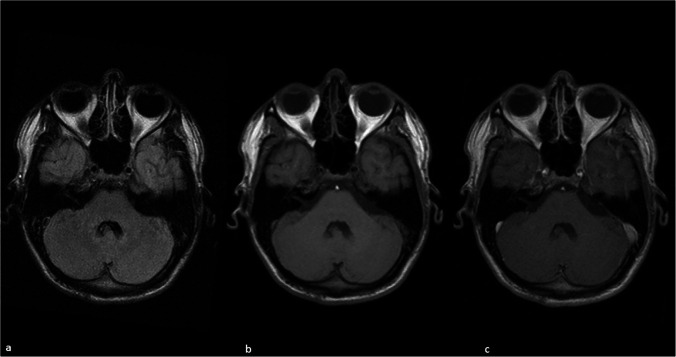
Unremarkable MRI by the time of first appearance (a) axial T2 FLAIR imaging (b) pre-KM T1 weighted imaging (c) post-KM T1 weighted imaging at the level of cerebellopontine peduncle
